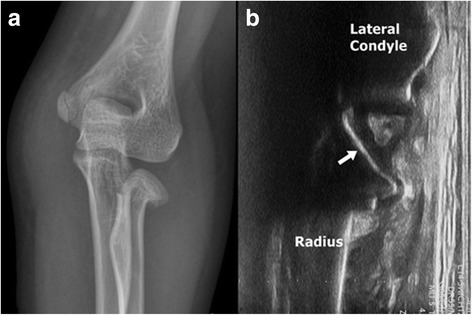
Radiographic and ultrasonographic views of the radial neck fracture. a Radial neck fracture Judet grade 3. b Intraoperative ultrasonographic view of the fracture. The reverberating echo of the angulated radial head (arrow) is clearly visible
